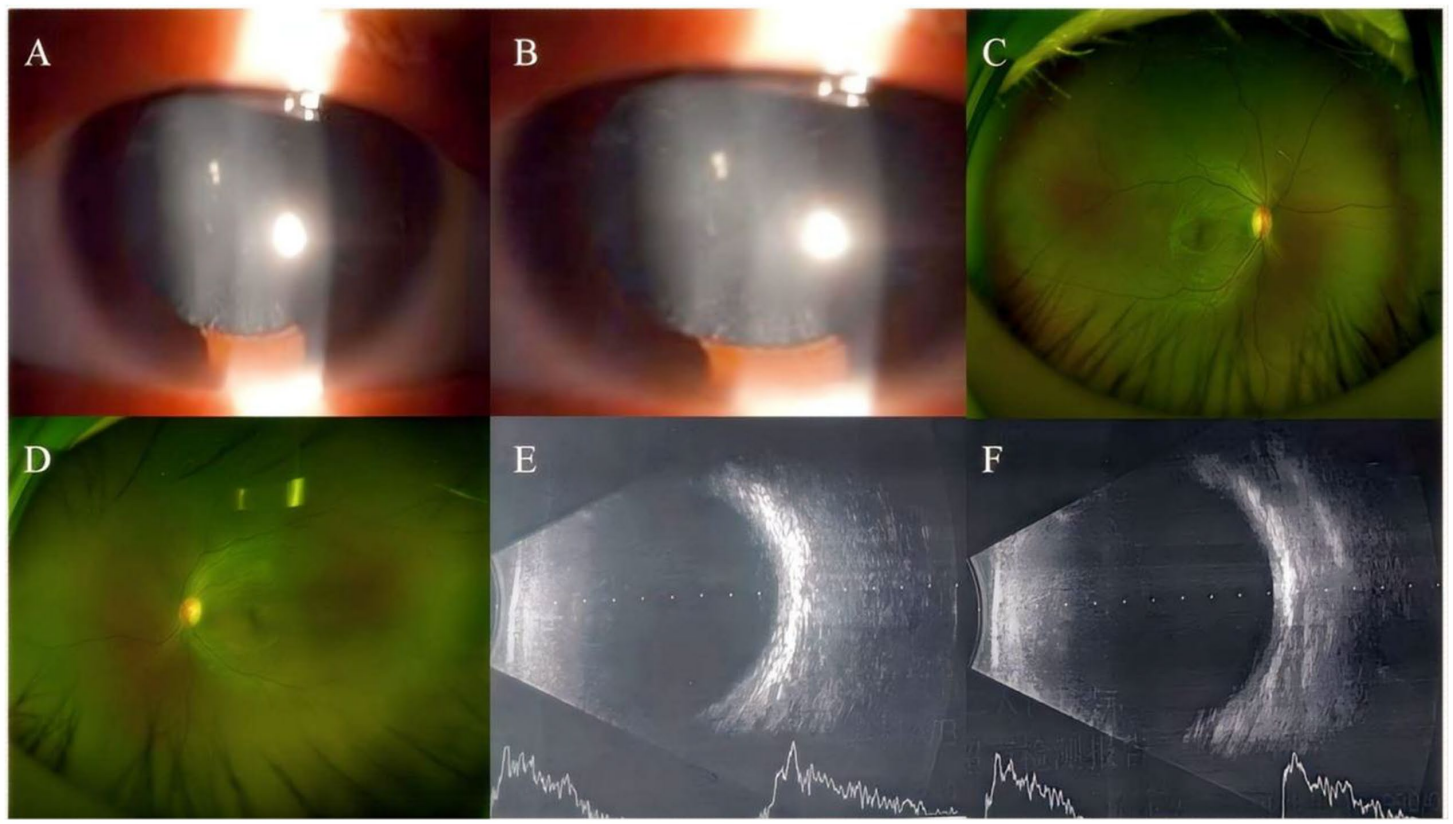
Anterior segment imaging. Anterior segment examination in the left eye of the proband reveals central cortical punctate opacities in the lens (A,B). Ultra-widefield fundus imaging. Bilateral ultra-widefield fundus images of the proband demonstrate sharp margined optic disc with normal coloration, visible macular reflex, and attached retina (C: right eye, D: left eye). B-scan ultrasonography. Bilateral B-scan ultrasonography shows clear vitreous body and attached retina in both eyes (E: right eye, F: left eye).
